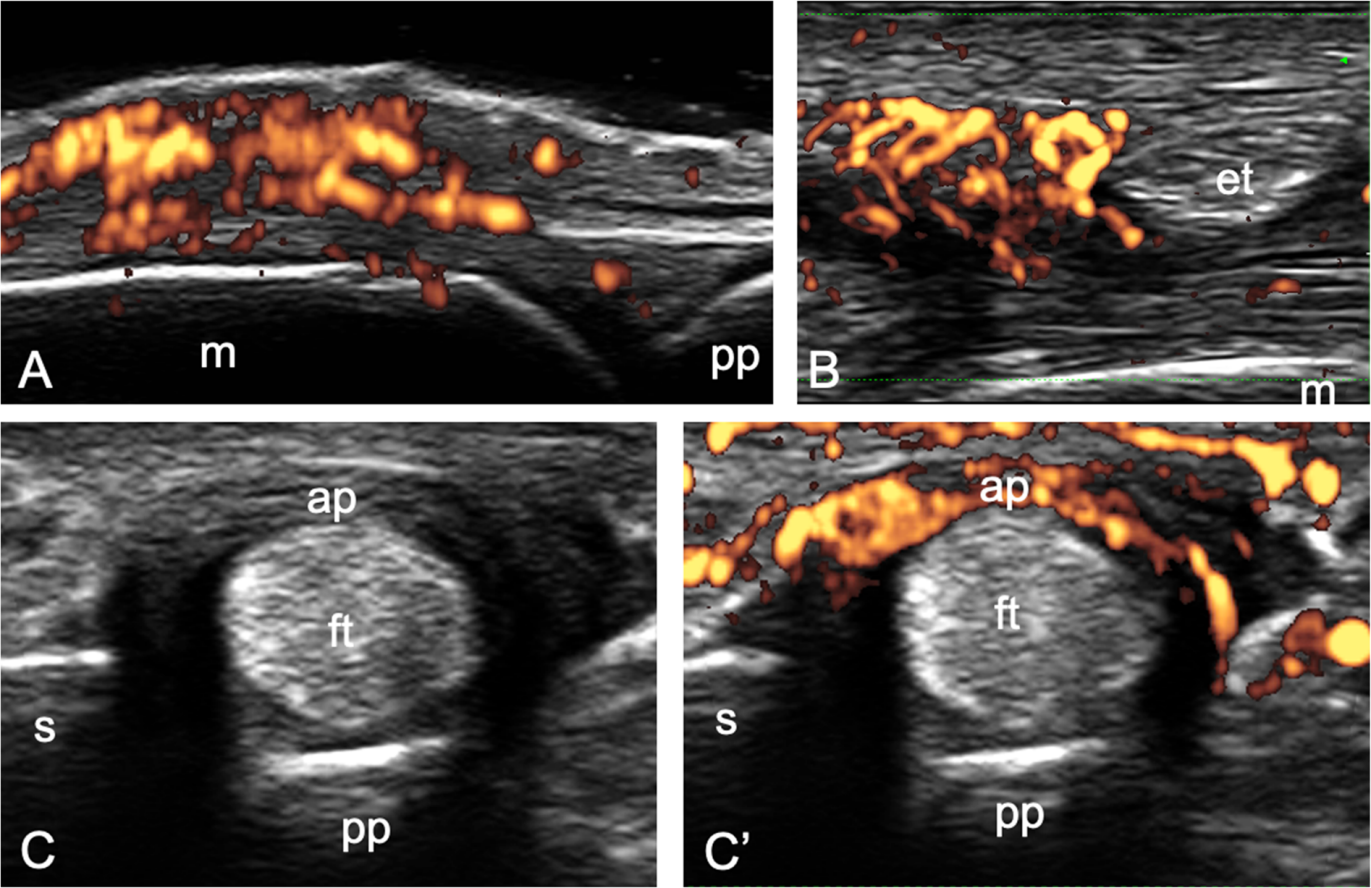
Functional enthesitis in psoriatic arthritis. In A and B, longitudinal and transverse scans obtained at the dorsal aspect of a metacarpophalangeal joint with 6–18-MHz and 22-MHz probes show a peritenon extensor tendon inflammation (PTI) pattern. In C and C’, transverse scans obtained at the volar aspect of a metacarpophalangeal joint of another patients with a 22-MHz probe show A1 pulley (ap) inflammation (power Doppler signal inside a thickened pulley). et = extensor tendon, ft = finger flexor tendons, m = metacarpal bone, pp = proximal phalanx, s = sesamoid bone
